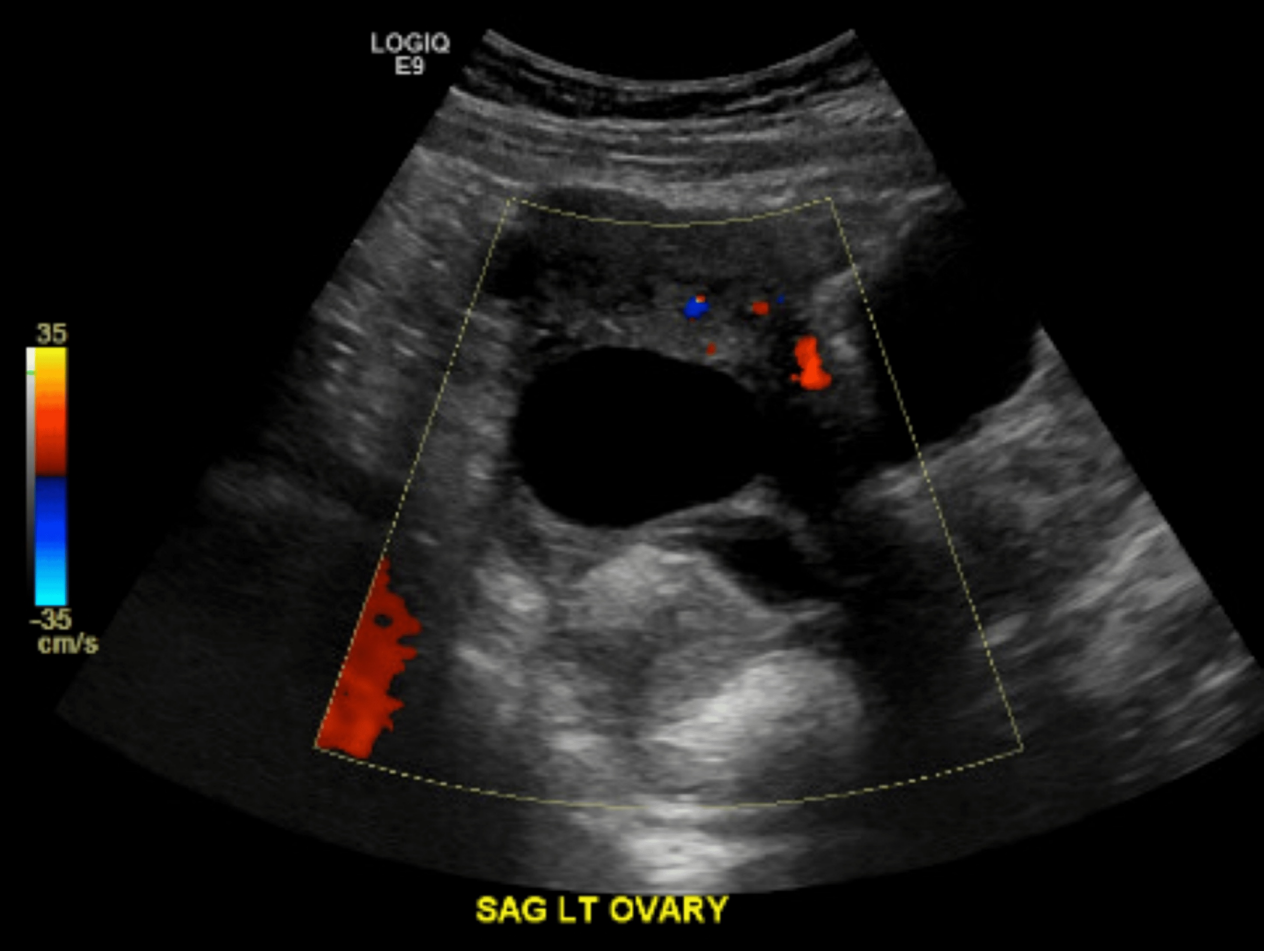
Ultrasound sagittal view of the left ovary This ultrasound report shows a sagittal view of the patient's left ovary measuring 9.3 × 5.5 × 9.8 cm, which is significantly larger than the right ovary, measuring 2.6 × 1.9 × 1.9 cm. There is a 4.2 cm cystic focus in the left ovary and a 7.7 cm left ovarian lesion, which was stated with uncertainty in the radiology report as a possible endometrioma.
